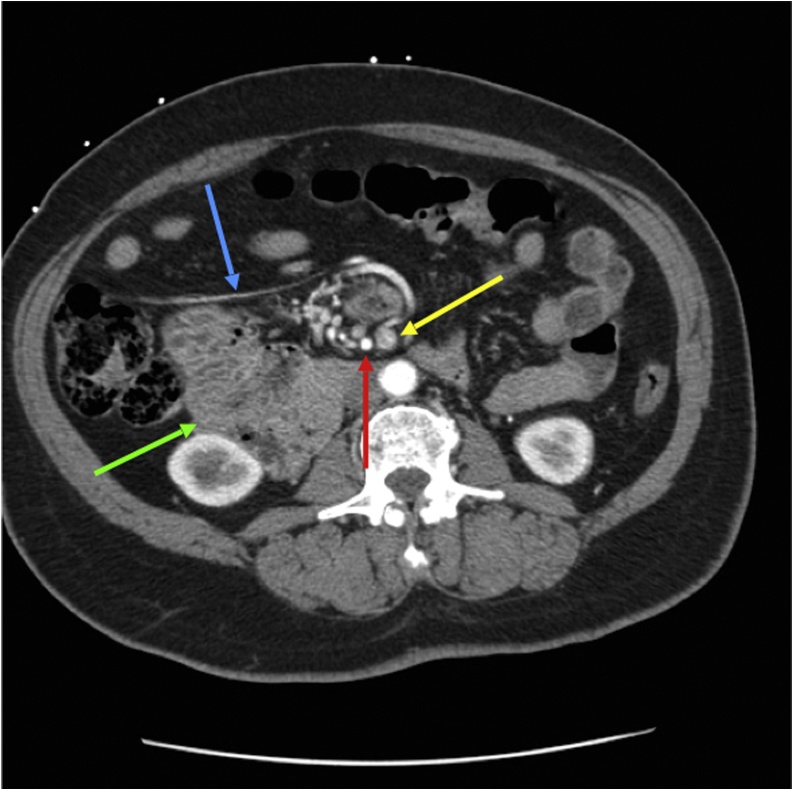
Axial image of prior contrast-enhanced CT Abdomen and Pelvis obtained in 2009, nine years prior to this surgery. Axial image in early arterial phase demonstrating reversal of superior mesenteric artery (red arrow) and superior mesenteric vein (yellow arrow). Abnormal linear configuration of possible vessel or Ladd band seen in the right upper quadrant (blue arrow). Third and fourth portions of duodenum (green arrow) abnormally seen in the right hemi-abdomen anterior to right kidney and medial to the proximal large bowel, as opposed to their regular configuration in the left hemi-abdomen.
